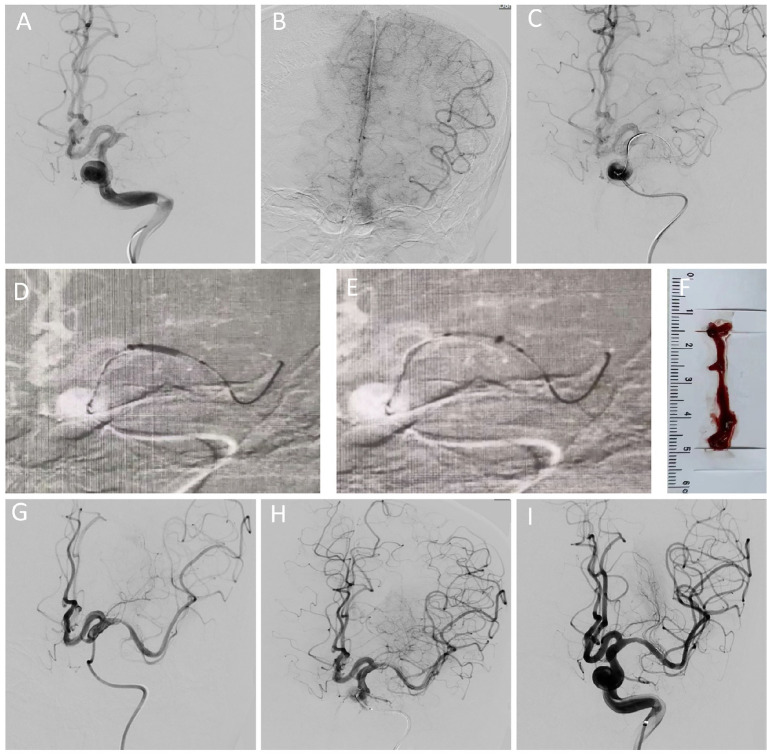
Patient presented with sudden right-sided limb weakness and loss of consciousness, with the last known normal time being 5 h prior to admission. Initial head CT from an outside facility showed no signs of hemorrhage. (A,B) Cerebral angiography revealed occlusion of the left middle cerebral artery with a tapered appearance and partial meningeal collateral circulation compensation in the late arterial phase. (C) Confirmation of distal true lumen patency after passage through the lesion site using a microguidewire and microcatheter, with a positive initial pass and a significant thrombus seen post-narrowing. (D,E) Cross-passage performed at the stenotic lesion site using the BAT technique. (F,G) Significant thrombus clearance was achieved at the distal lesion end during aspiration; follow-up imaging showed severe narrowing at the M1 segment of the middle cerebral artery. (H,I) Effective vessel remodeling following balloon dilation for proximal stenosis; an Enterprise 4 * 23 mm stent was implanted in the M1 segment of the middle cerebral artery to achieve mTICI 3 reperfusion. Post-BAT and mechanical thrombectomy, no signs of intracranial hemorrhage or extensive cerebral infarction were observed.
